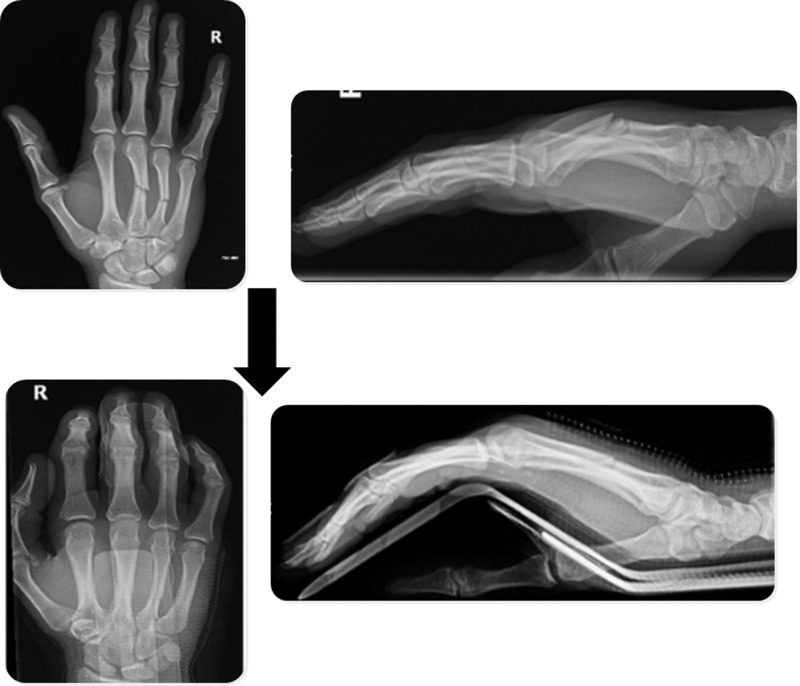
Radiographs demonstrating initial middle and ring displaced metacarpal fractures and radiographs at three-weeks after closed reduction, with splint
